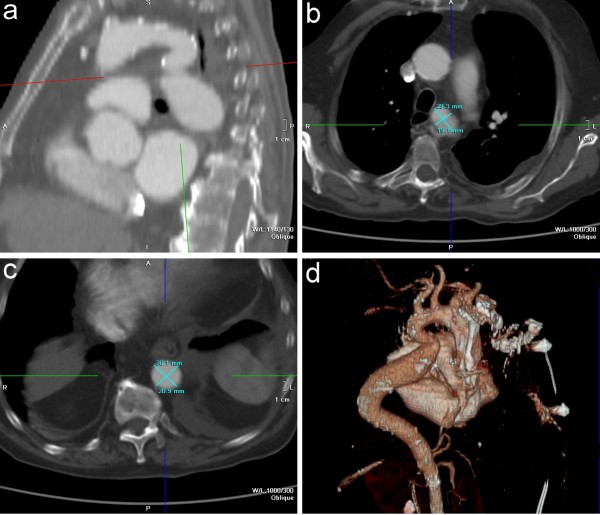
Computed Tomography angiography. 2D parasagittal scan view of CoA (a); 2D CoA diameters (b), 2D aortic diameters at the diaphragm level (c); 3D volume-rendered reconstruction of the thoracic aorta showing significant tortuosity and no developed collateral circulation (d).
